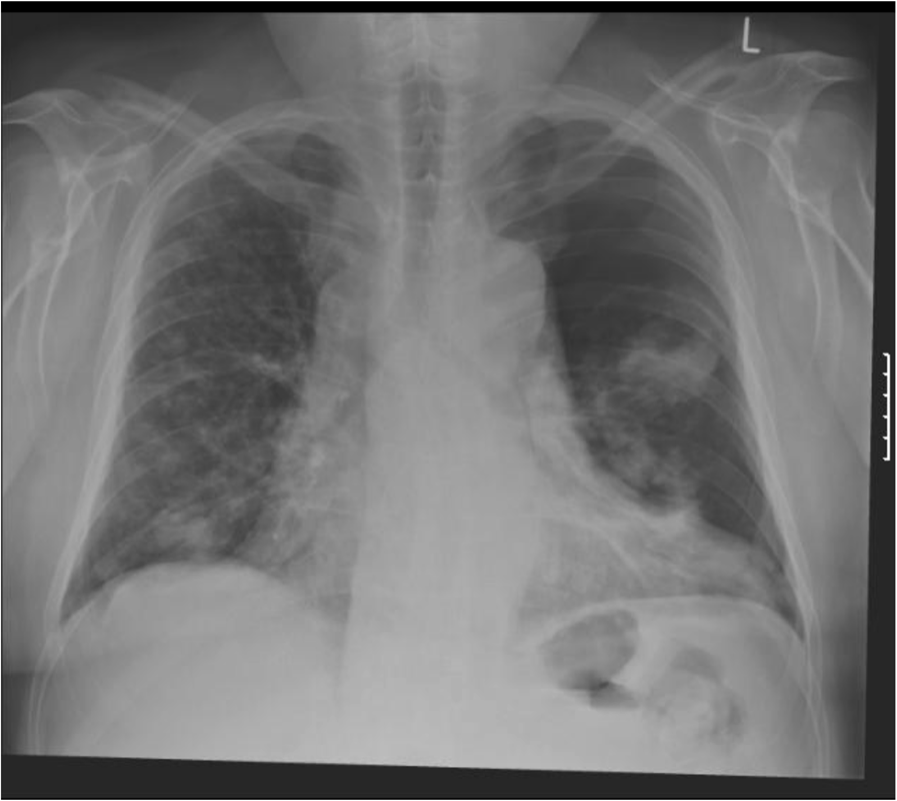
Chest radiograph showing a 50% left-sided pneumothorax. 50% left-sided pneumothorax as visualised on a simple chest radiograph following 4 weeks of crizotinib treatment.
